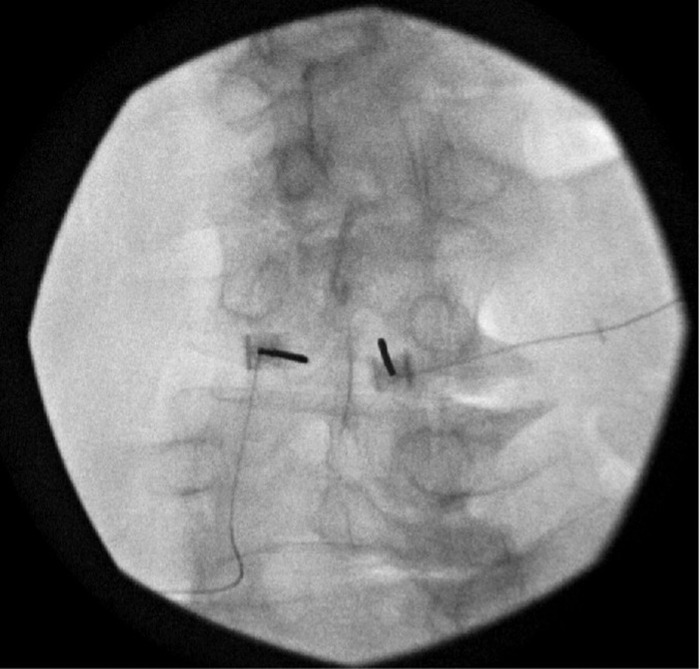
Radiographic image of the introducer placement for the SPRINT peripheral nerve stimulation device at the level of L4 bilaterally, targeting the median nerves for the patient described in case 3.
